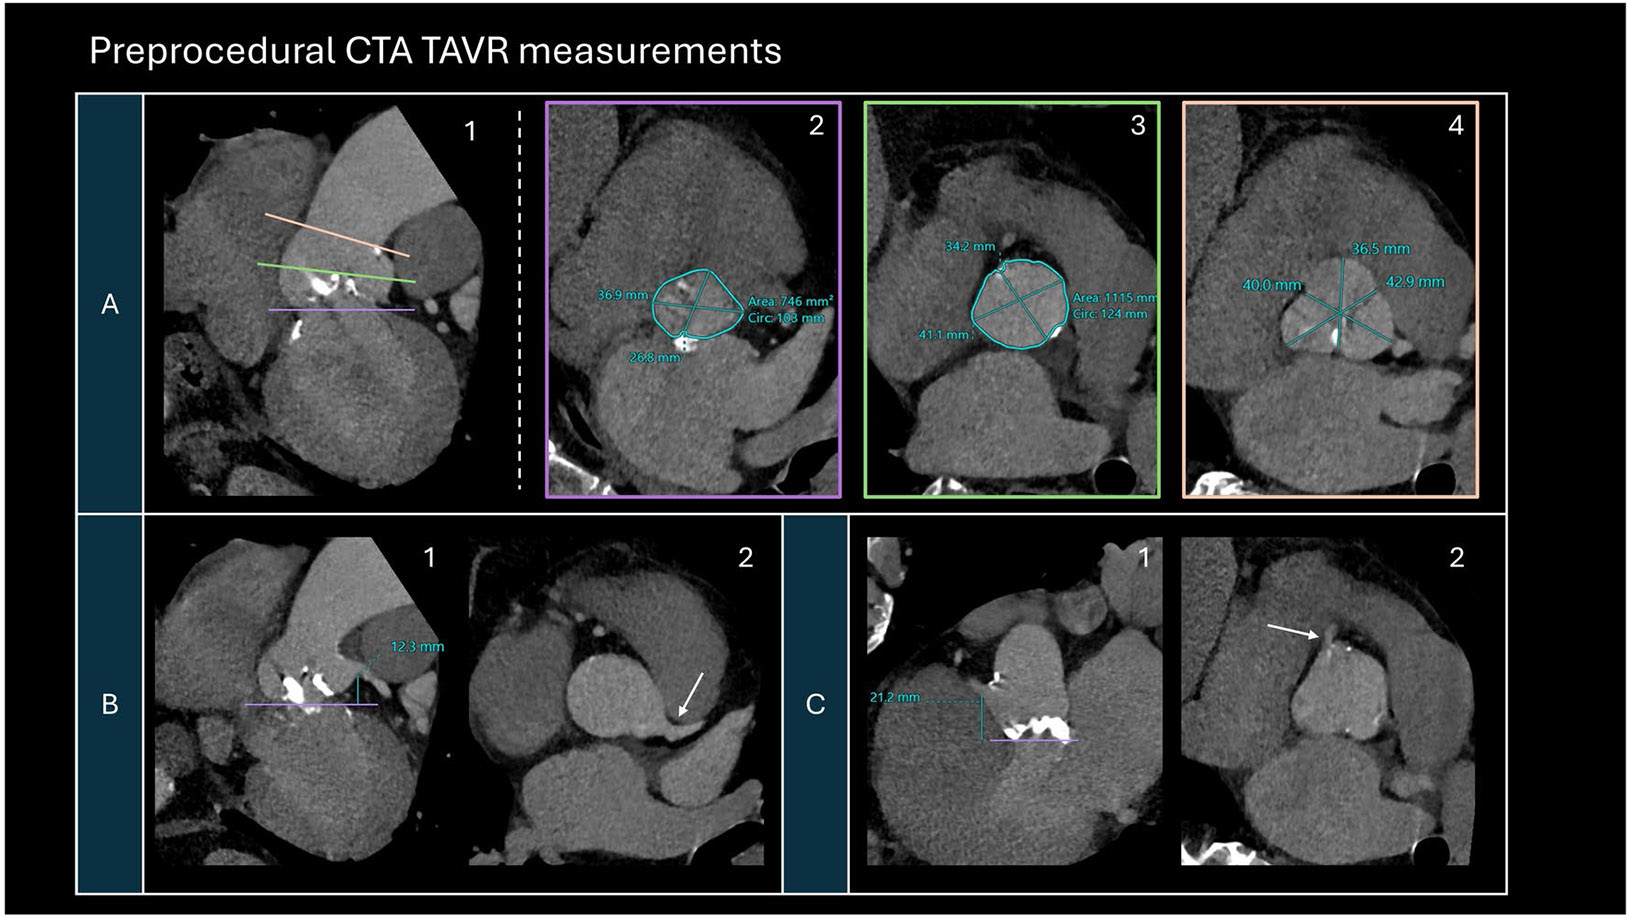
A representative sample of common preprocedural CTA TAVR measurements commonly performed for operative planning and valve selection. A Multiplanar double oblique images of the aortic root along the long axis (A1) with levels (solid lines) corresponding to the true short axis views of the aortic annulus (A2), sinotubular junction (A3), and Sinuses of Valsalva (A4). B Measurement of the coronary ostia height of the left main coronary artery at the takeoff from the left coronary cusp (arrow) as seen on the longitudinal (B1) and the short axis (B2) views. C Measurement of the coronary ostia height of the right coronary artery at the takeoff from the right coronary cusp (arrow) as seen on the longitudinal (C1) and the short axis (C2) views
